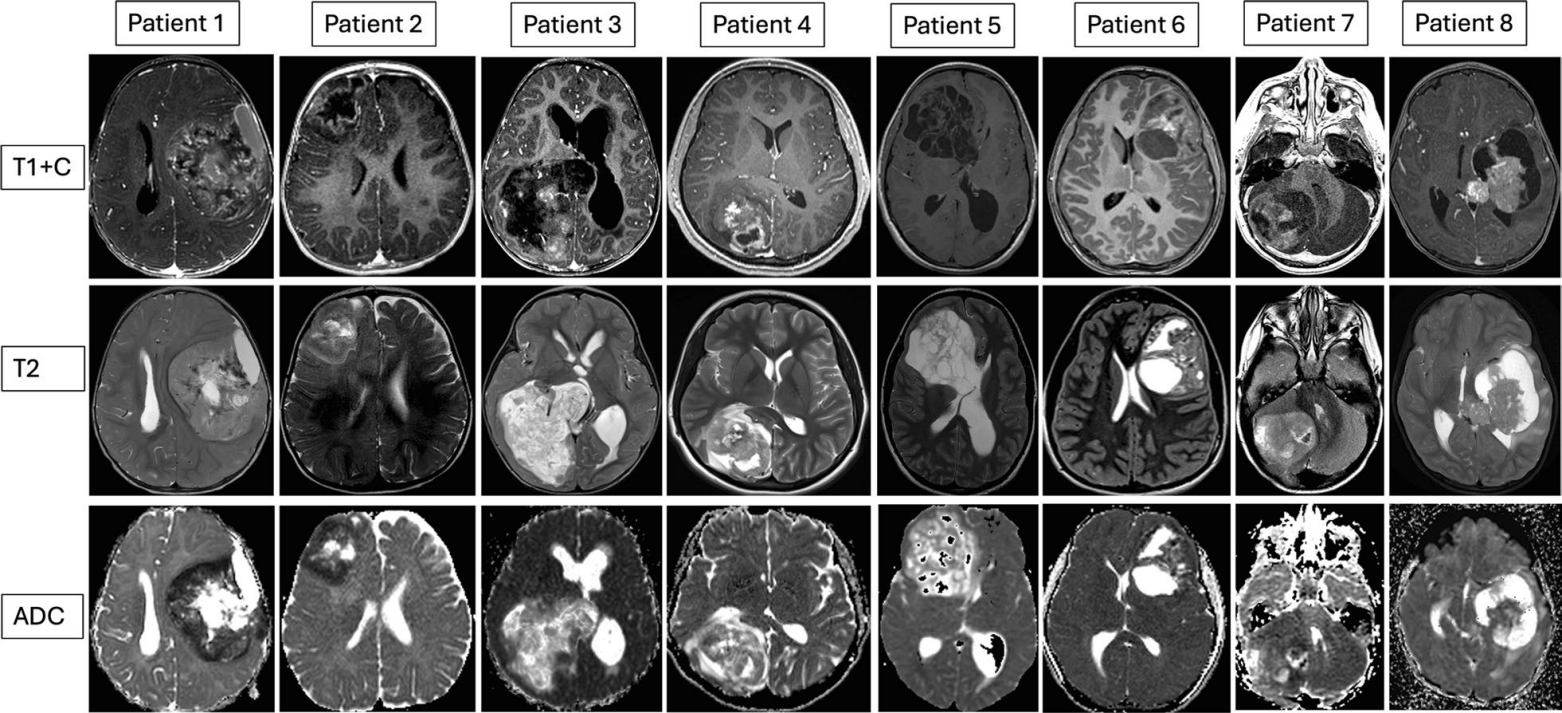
Representative pre-operative brain MRI images of neuroepithelial tumors with PLAG-family genetic alterations are shown. Cases #1–6 have *EWSR1-PLAGL1* rearrangement, while Case #7 and #8 have *PLAGL2* amplification or *PLAGL1* amplification respectively. The top row demonstrates post-contrast T1-weighted sequences, the middle row demonstrates T2-weighted sequences, and the bottom row shows apparent diffusion coefficient maps. Heterogeneous enhancement and diffusion restriction of the solid component are the most common findings, except for tumor #5, which was multicystic and showed no enhancement or diffusion restriction. Tumor cysts were present in case #5, 6, and 8. Additional imaging details are available in supplementary Table 1
